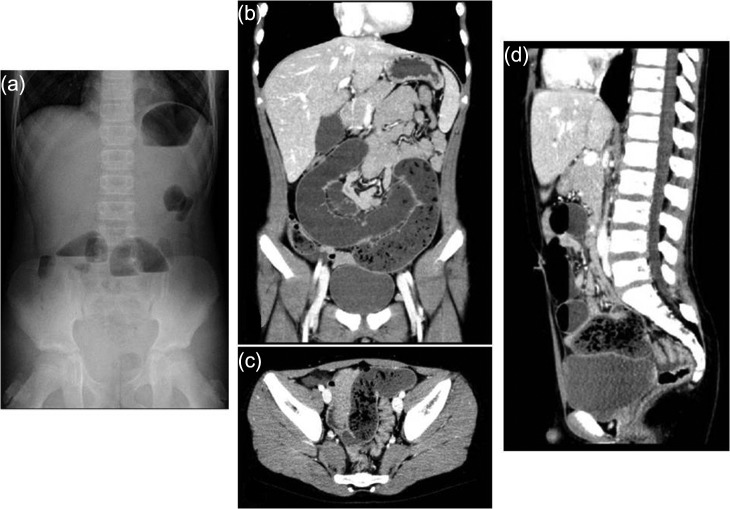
Abdominal X-ray and enhanced computed tomography. (a) Abdominal X-ray on admission showing multiple air-fluid levels. (b–d) Enhanced computed tomography showing small bowel feces sign, which is defined as the presence of feces-like material in the lumen of dilated loops of small bowel proximal to the site of obstruction.
